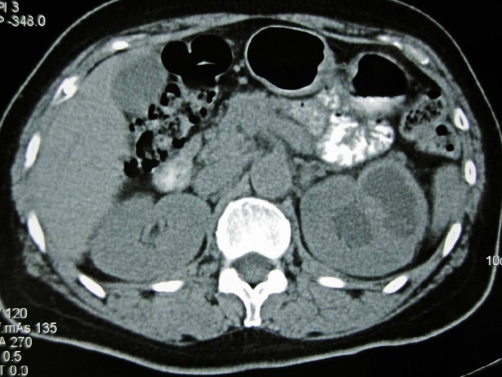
Plain CT image demonstrates a cystic mass lesion in the upper pole of the left kidney. No calcifications were detected.
